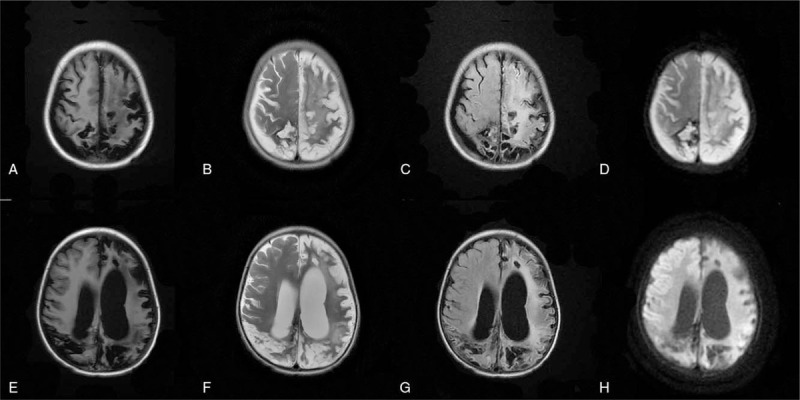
Head MRI 5 months after surgery. Note: A and E are T1WI, B and F are T2WI, C and G are FLAIR, and D and H are DWI. DWI = diffusion-weighted imaging, FLAIR = fluid attenuated inversion recovery, MRI = magnetic resonance imaging, T1WI = T1 weighted image, T2WI = T2 weighted image.
